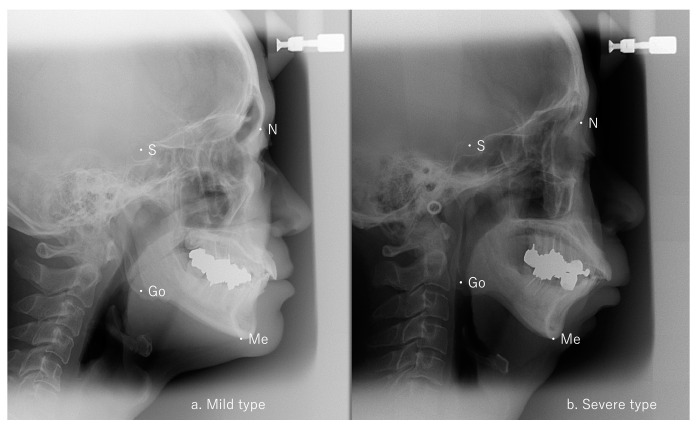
Lateral cephalometric radiographs. (a) Mild AHI patient: S-Go/N-Me 65.6% and Go-Me/S-N 102.1%, (b) severe AHI patient: S-Go/N-Me 67.5% and Go-Me/S-N 99.8%, AHI: apnea–hypopnea index.
